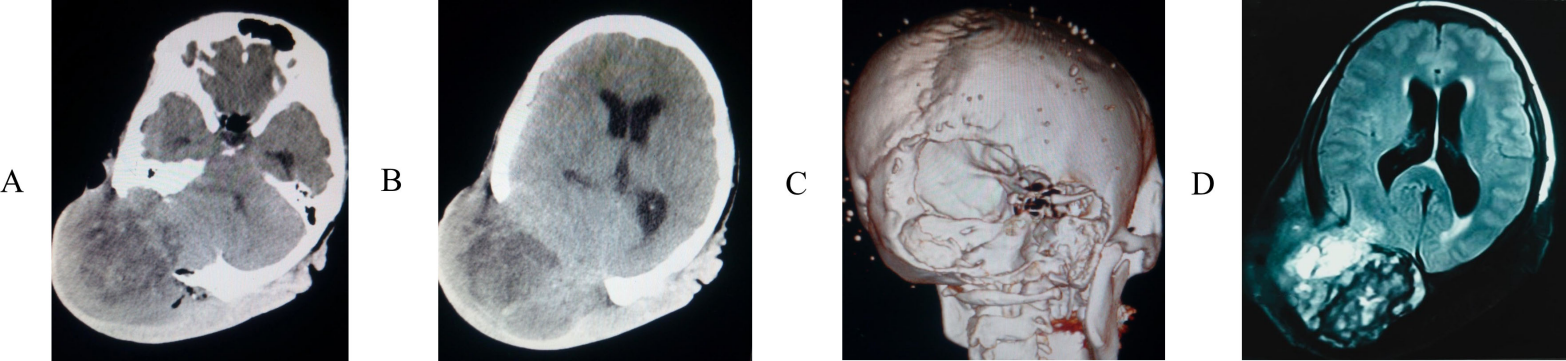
The computed tomography (CT) scan (A, B) showed that the tumor located on the right side of the occipital and vertex region, 3D reconstruction (C) revealed the skull was severely destroyed. (D) Magnetic resonance imaging (MRI) revealed the tumor signal was mixed and that neighboring brain tissue was compressed, poorly circumscribed lesion with infiltrative border.
